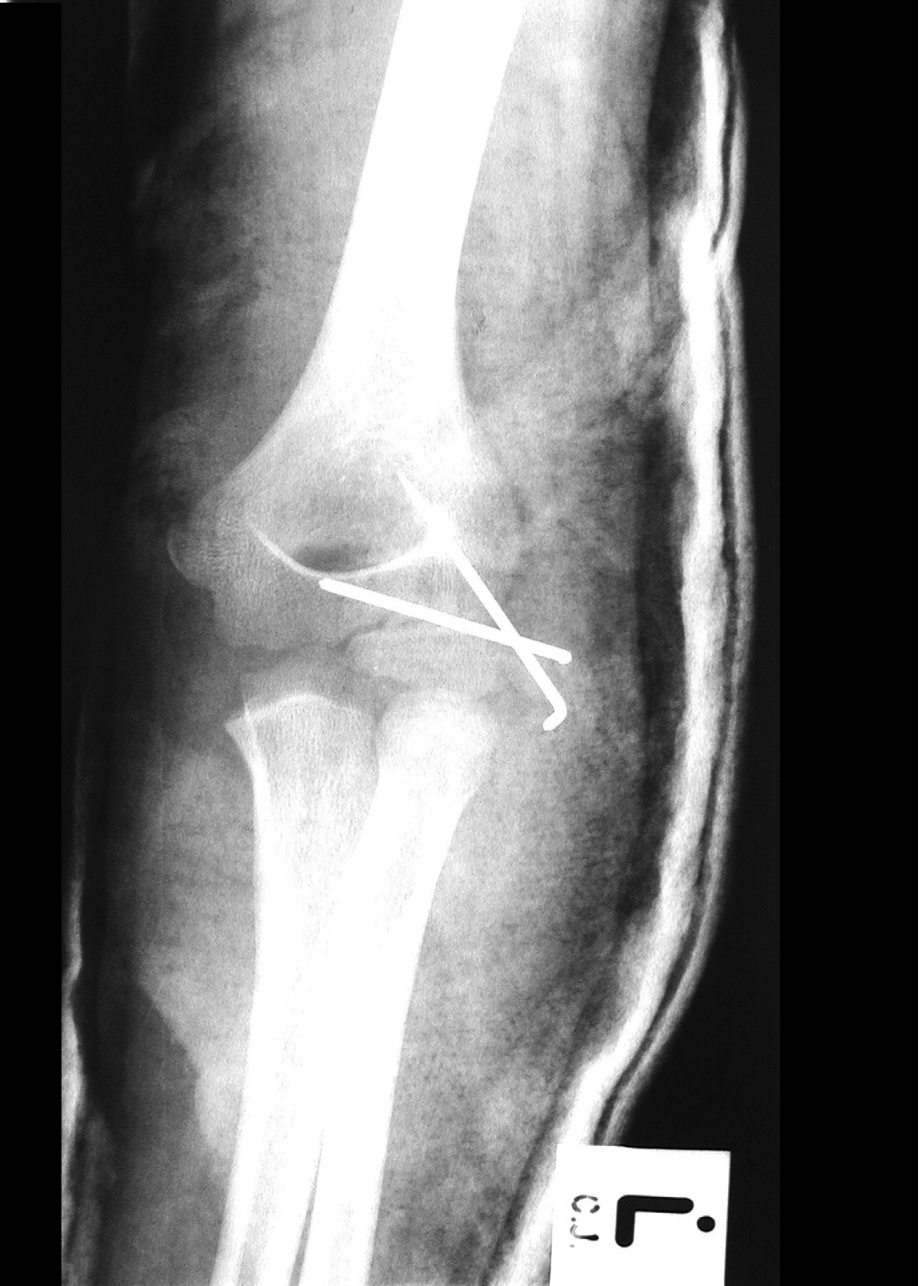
Postoperative anteroposterior radiograph of the elbow revealing internal fixation of the lateral condyle fracture with the help of 2 K-wires. The elbow dislocation was reduced first by closed technique.
